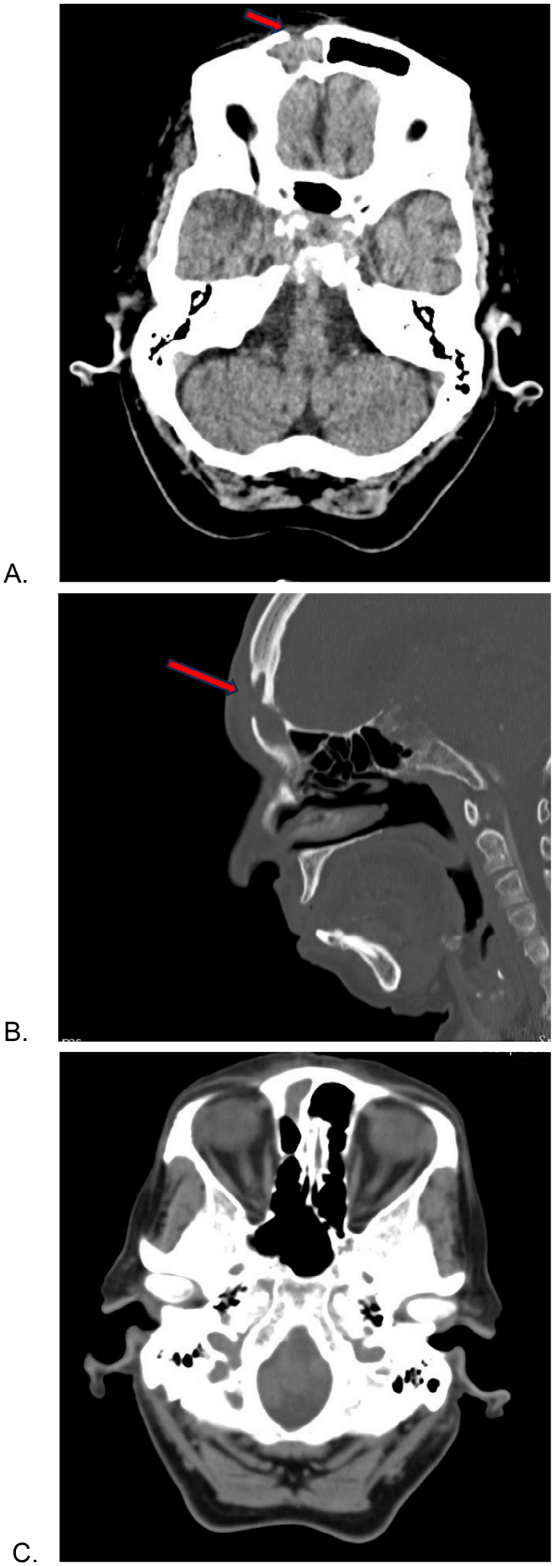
Computed tomography in patient (A–C). Right frontal sinus is completely opacified (A). A focal bony defect is visualized in the anterior wall of the right frontal sinus (arrows) consistent with prior craniotomy. There is also evidence of osteomyelitis and soft tissue swelling of the forehead. No intracranial hemorrhage, mass lesions, or radiographic evidence of cerebral infarction is identified. Opacification extends to the ethmoidal sinus (C).
